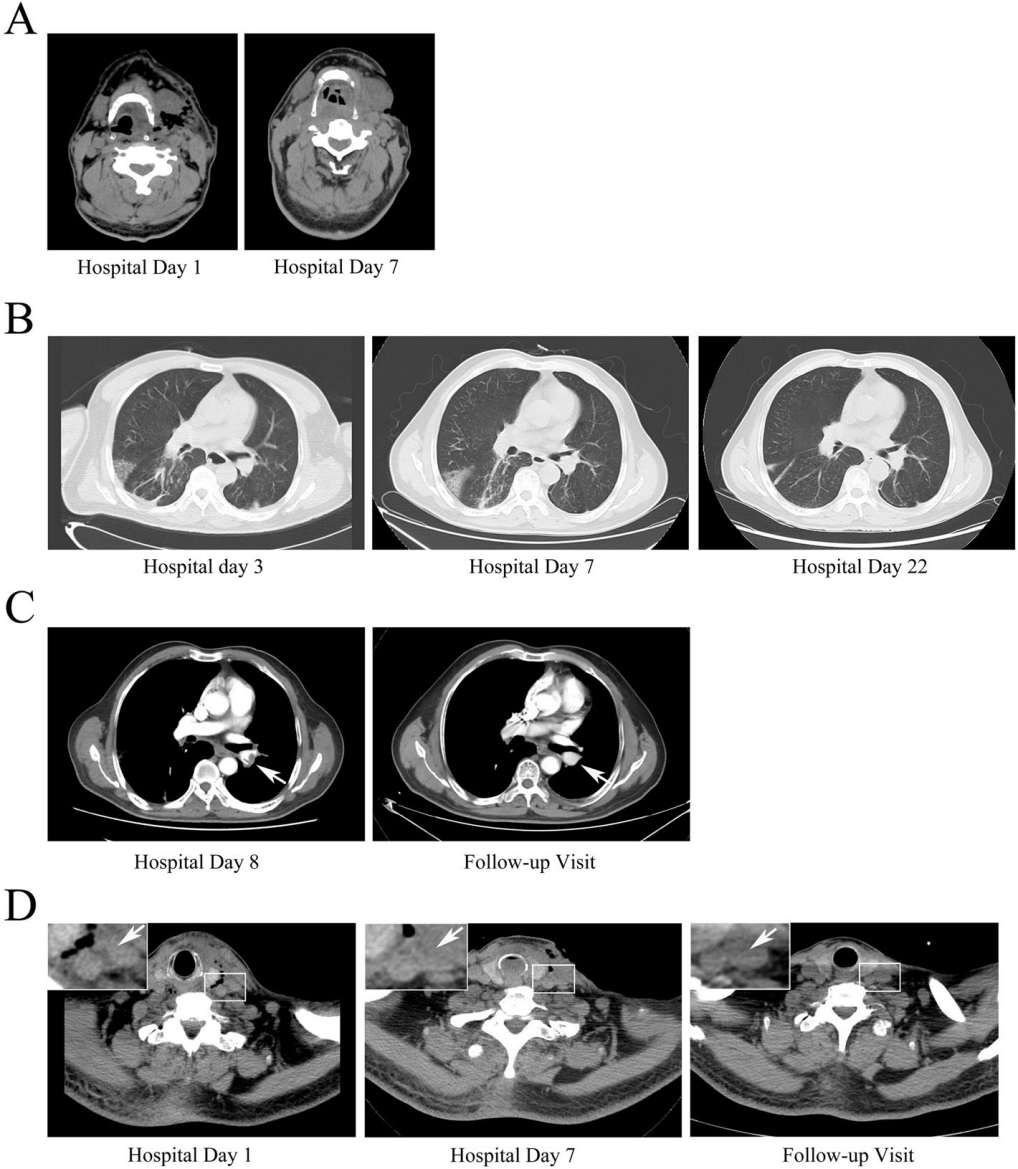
Imaging. A: Neck CT. B: Chest CT. C: Pulmonary artery CT. White arrow: pulmonary emboli in the “Hospital Day 8” image; pulmonary emboli disappeared in the “Follow-up Visit” image. D: Internal jugular vein CT. White arrow: signs of inflammation next to the internal jugular vein in “Hospital Day 1” and “Hospital Day 7” images; the signs of inflammation disappeared in the “Follow-up Visit” image
